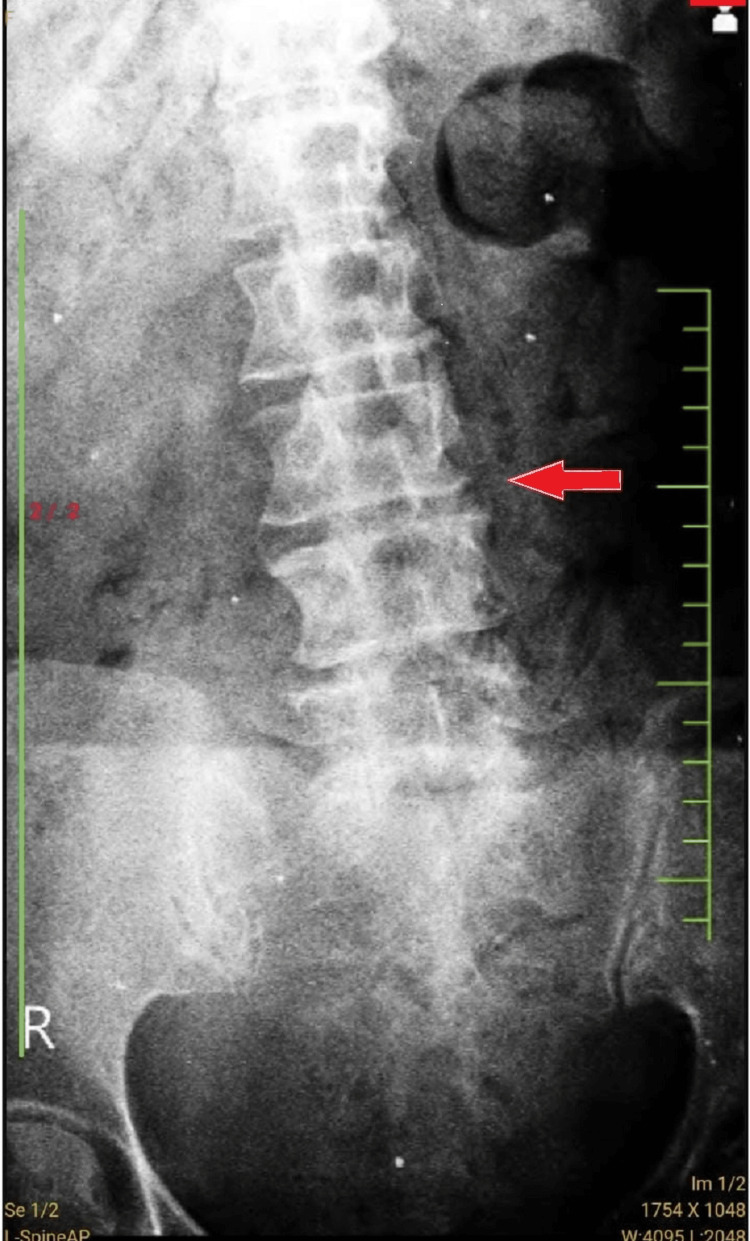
Shows the X-ray of lumbar compression at level L3 to S1 level (red arrow) L - lumbar, S - sacral
